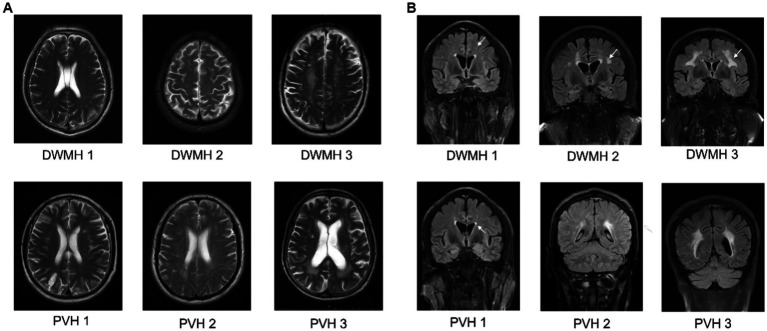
The transverse (A) and coronal (B) sections of MRI of Fazekas scale to semi-quantitatively quantify the WMLs severity. For deep white matter hyperintensity (DWMH): punctate foci, score 1; small confluences, score 2; large confluences, score 3. For periventricular hyperintensity (PVH): caps or pencil-thin linings, score 1; smooth halos with diameters of 6 mm ~ 10 mm, score 2; irregular lesions with diameters of more than 10 mm, score 3. DWMH, deep white matter hyperintensity; PVH, periventricular hyperintensity.
